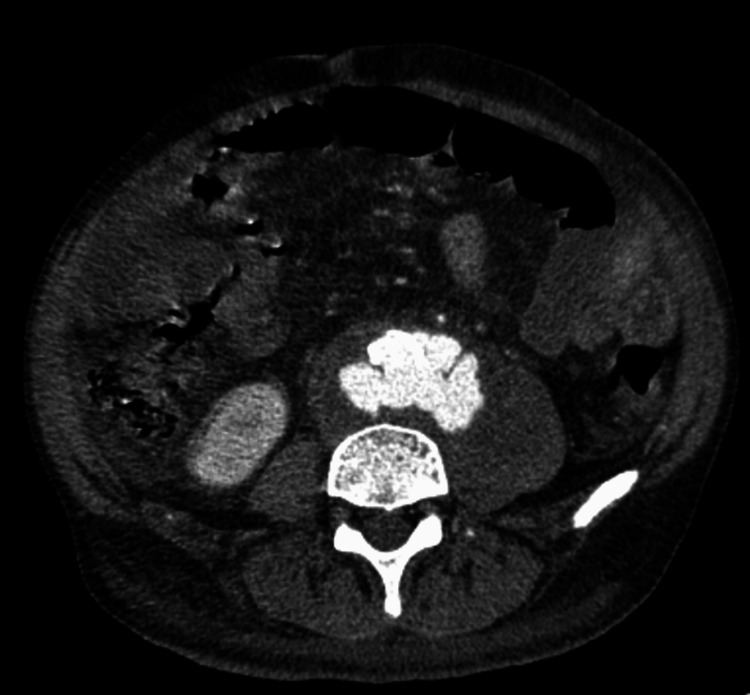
Abdominal computed tomography (CT) imaging with contrast. The pseudoaneurysm of the abdominal aorta appears markedly enlarged and in a state of imminent rupture. The abscess around the lesion and within the left iliopsoas and psoas major muscles with gas appears to be enlarged.
